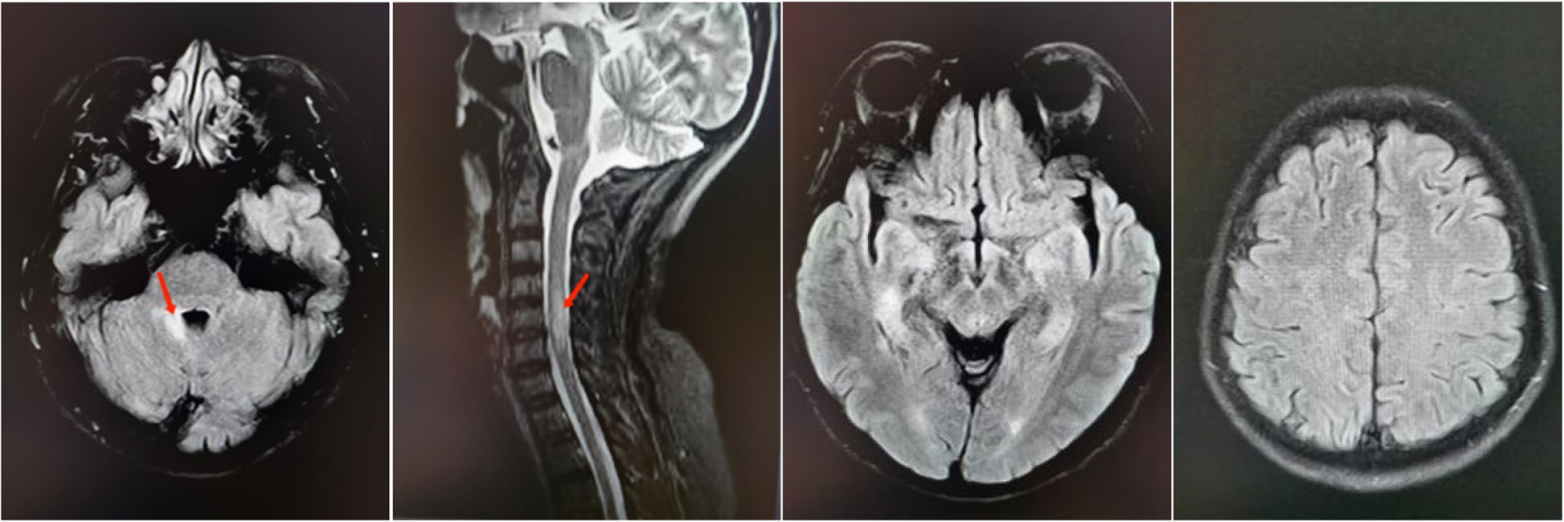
MRI shows T2 hyperintense at the dorsal pons near right surface of the fourth ventricle and spinal cord of the C4-C6 vertebrae,no lesions in bilateral occipital, parietal, and frontal lobes
